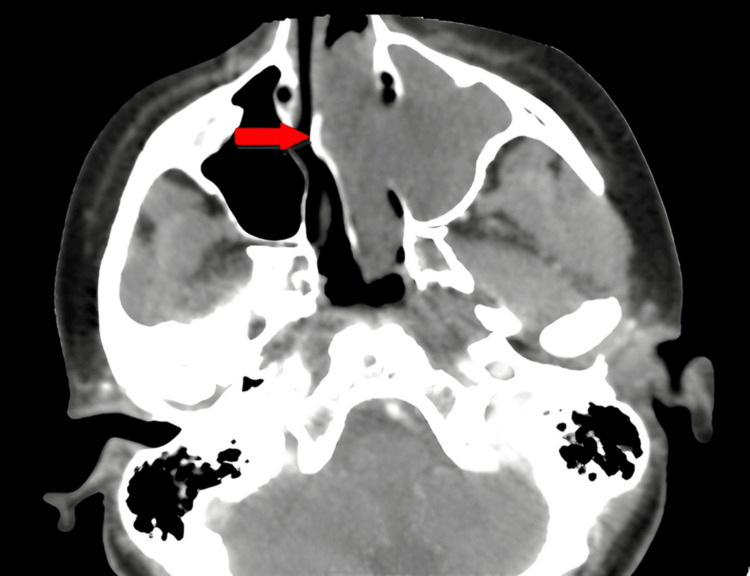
Non-injected axial CT scan: left antrochoanal polyp with septal deviation obstructing the right nasal cavity (red arrow)
